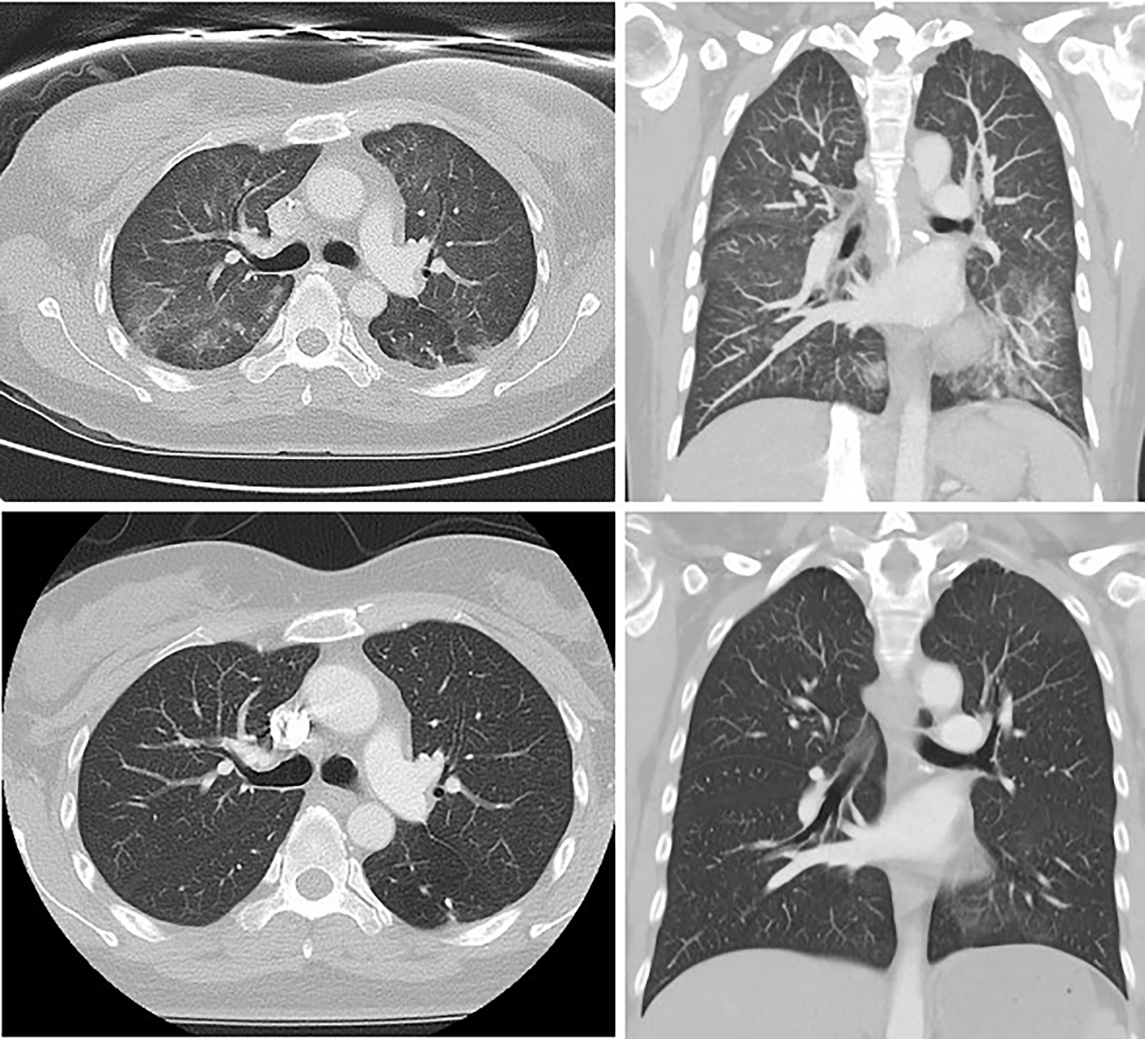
Thoracic HRCT from patient 4, demonstrating interstitial fibrosis, increased reticular markings, ground glass attenuation and traction bronchiectasis at the time of diagnosis (above) and subsequent standard-resolution thoracic CT showing resolution of these changes following 6 weeks of corticosteroids (below).
